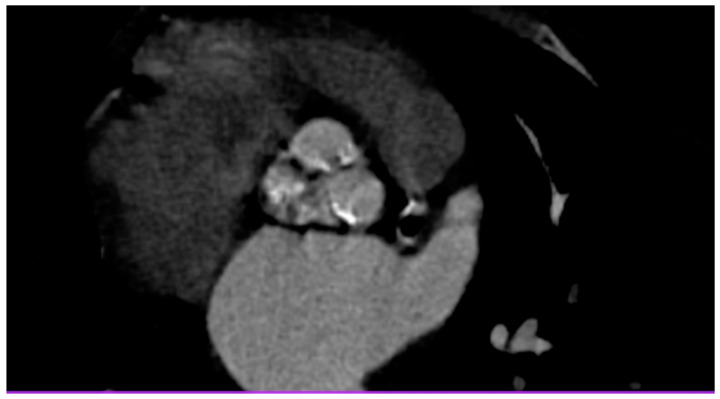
Calcified aortic valve (CT).
